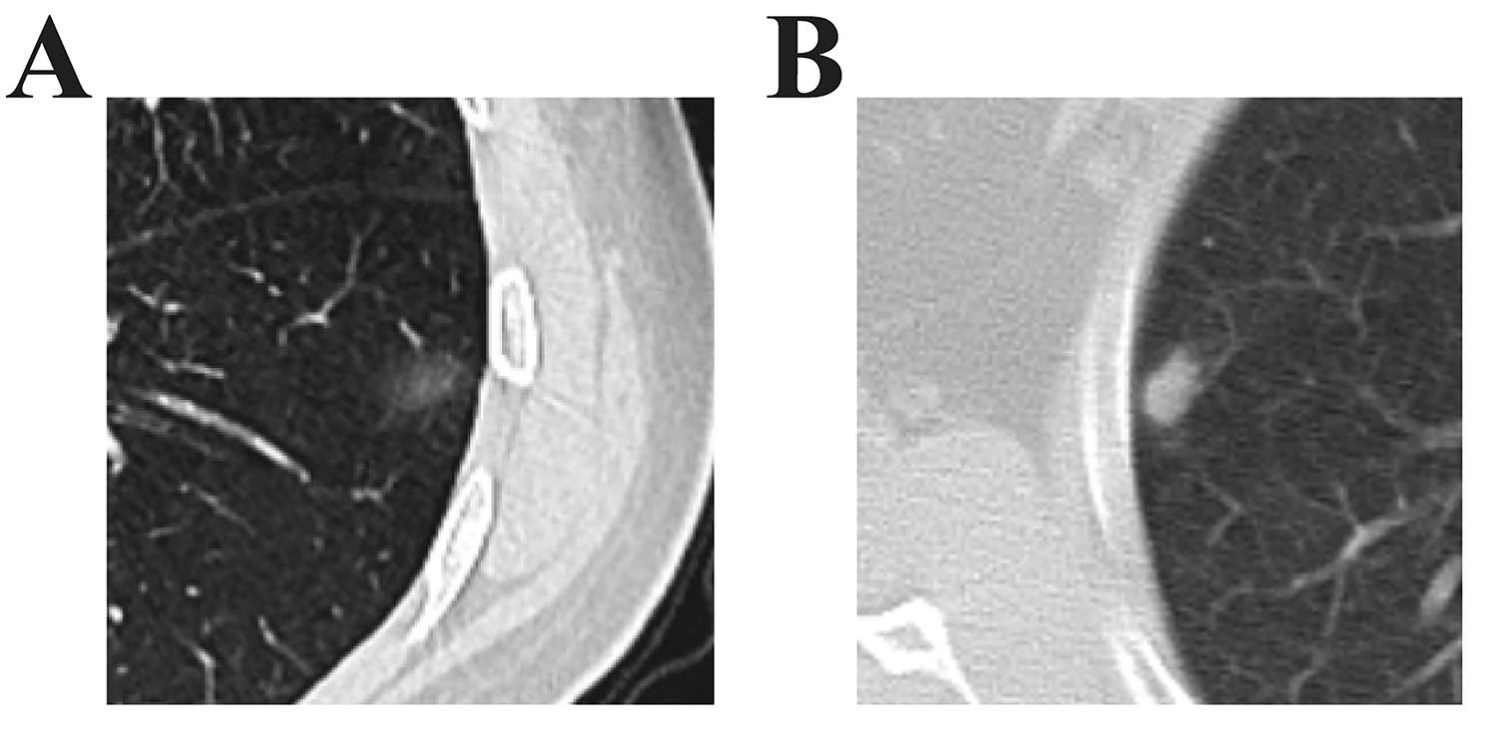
Image features of benign GGNs in Axial HRCT. (A) Chronic pneumonia in a 47-year-old female. HRCT shows a 14 mm focal ground-glass nodule with cloudy sign in the left lower lobe. (B) Granulomatitis with necrosis in a 63-year-old female. HRCT shows a 12.3 mm focal ground-glass nodule with homogenized patch sign in the right upper lobe, the mean CT attenuation value of nodule was − 221.90HU.
